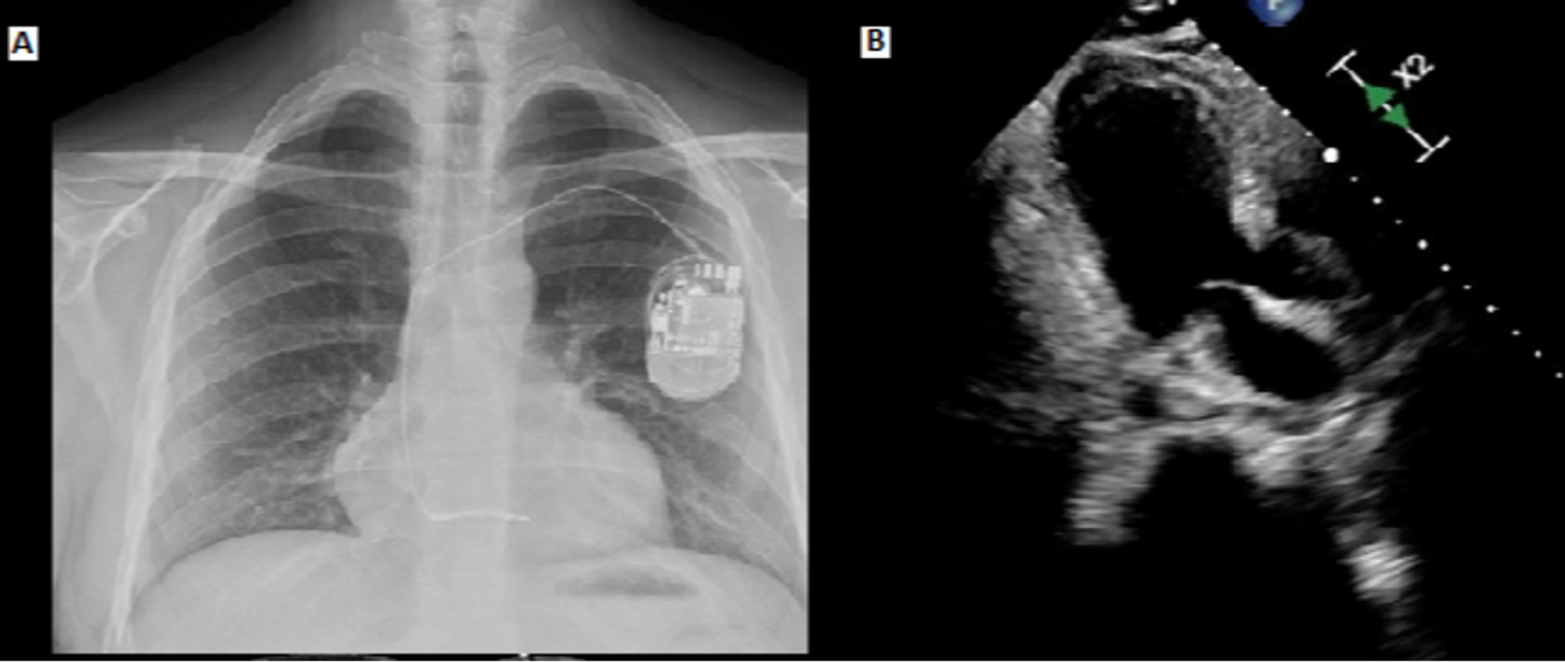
Cardiac imaging on admission A: chest radiograph demonstrated no acute cardiopulmonary disease. B: TTE - left ventricular function was severely globally reduced (20%); a repeat TTE performed one day later showed that left ventricular function had recovered TTE: transthoracic echocardiogram
